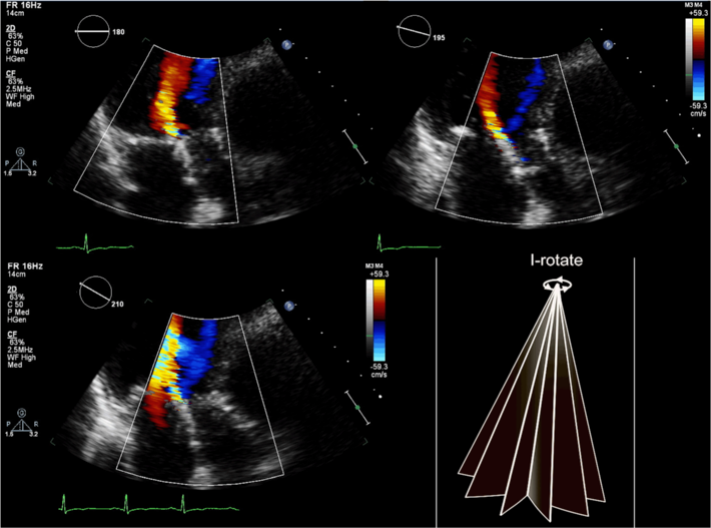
I-Rotate imaging in an apical window of PVL seen in a CoreValve prosthesis. Colour Doppler images are acquired at 15 degrees intervals
